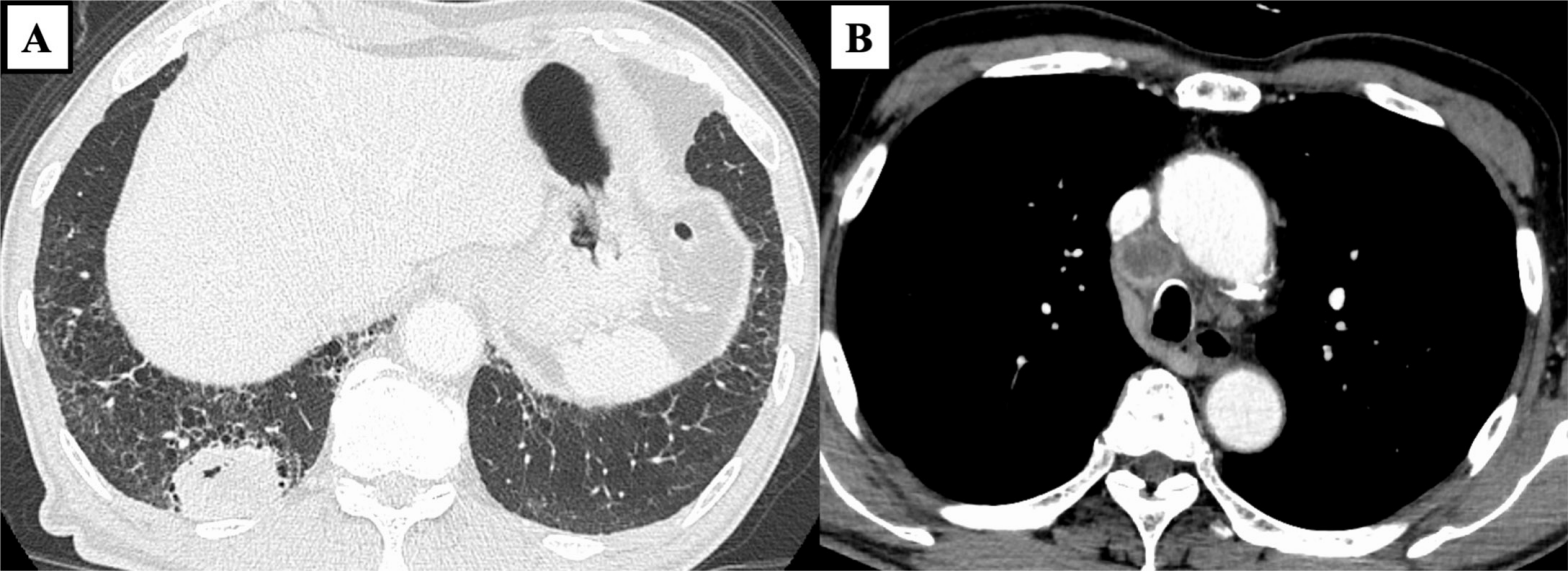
Chest computed tomography upon admission revealed a 45 mm tumour shadow in the right lower lobe of the lung (yellow arrows) and mediastinal lymph node enlargement (green arrow).
